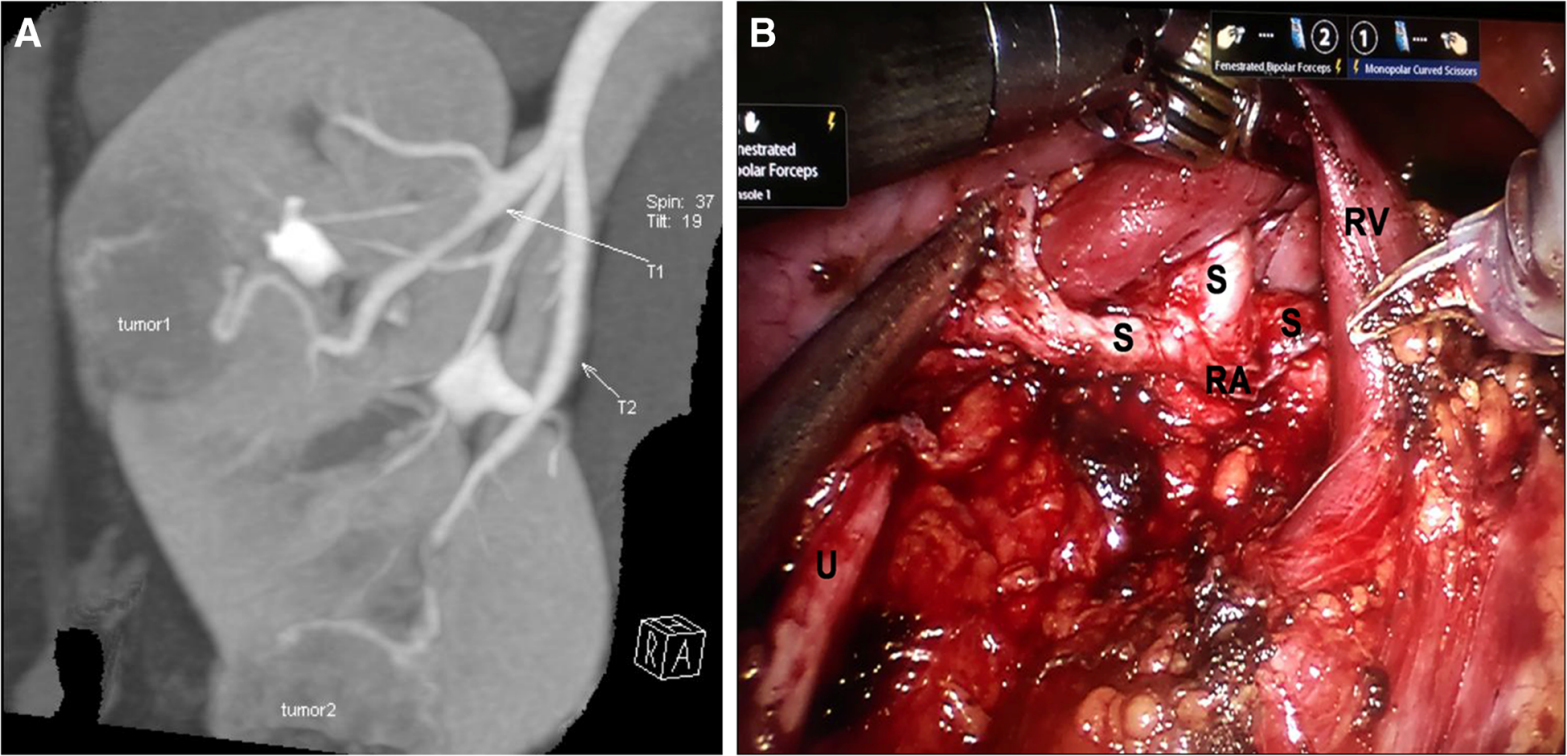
The preoperative picture (a) of dual-source computed tomography (DSCT) angiography precisely predicts the two segmental renal arteries (SRAs) (T1 and T2) feeding two different lesions (tumor1 and tumor2) in the right kidney respectively, as confirmed by the hilar anatomy during robot-assisted partial nephrectomy (RPN) (b). T = target SRA
